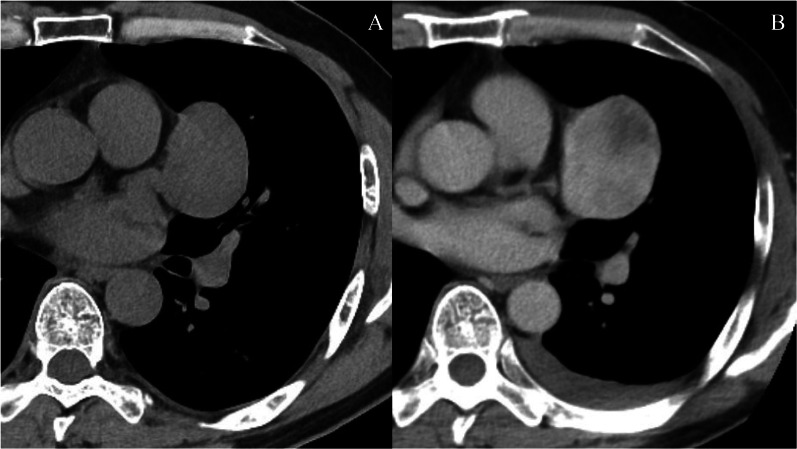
Chest CT images at the time of detection (A) and after tumor rupture (B). A Chest CT imaging showing a tumor in the left upper lobe of the lung. B Chest contrast-enhanced CT imaging showing a solid tumor (5 cm in diameter) close to the pericardium, and left pleural effusion. *CT* computed tomography
